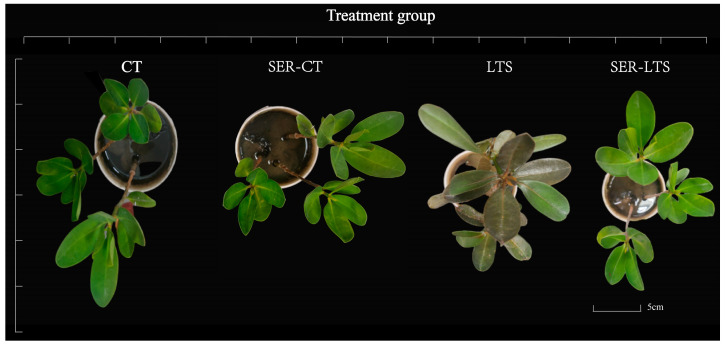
Serotonin pretreatment improves the cold resistance of *K. obovata* seedlings. Seedlings were grown under normal temperature conditions (25 °C/20 °C) without serotonin pretreatment (CT) or with serotonin pretreatment (SER-CT). Seedlings were grown under low-temperature conditions (6 °C/−3 °C) without serotonin pretreatment (LTS) or with serotonin pretreatment (SER-LTS).
